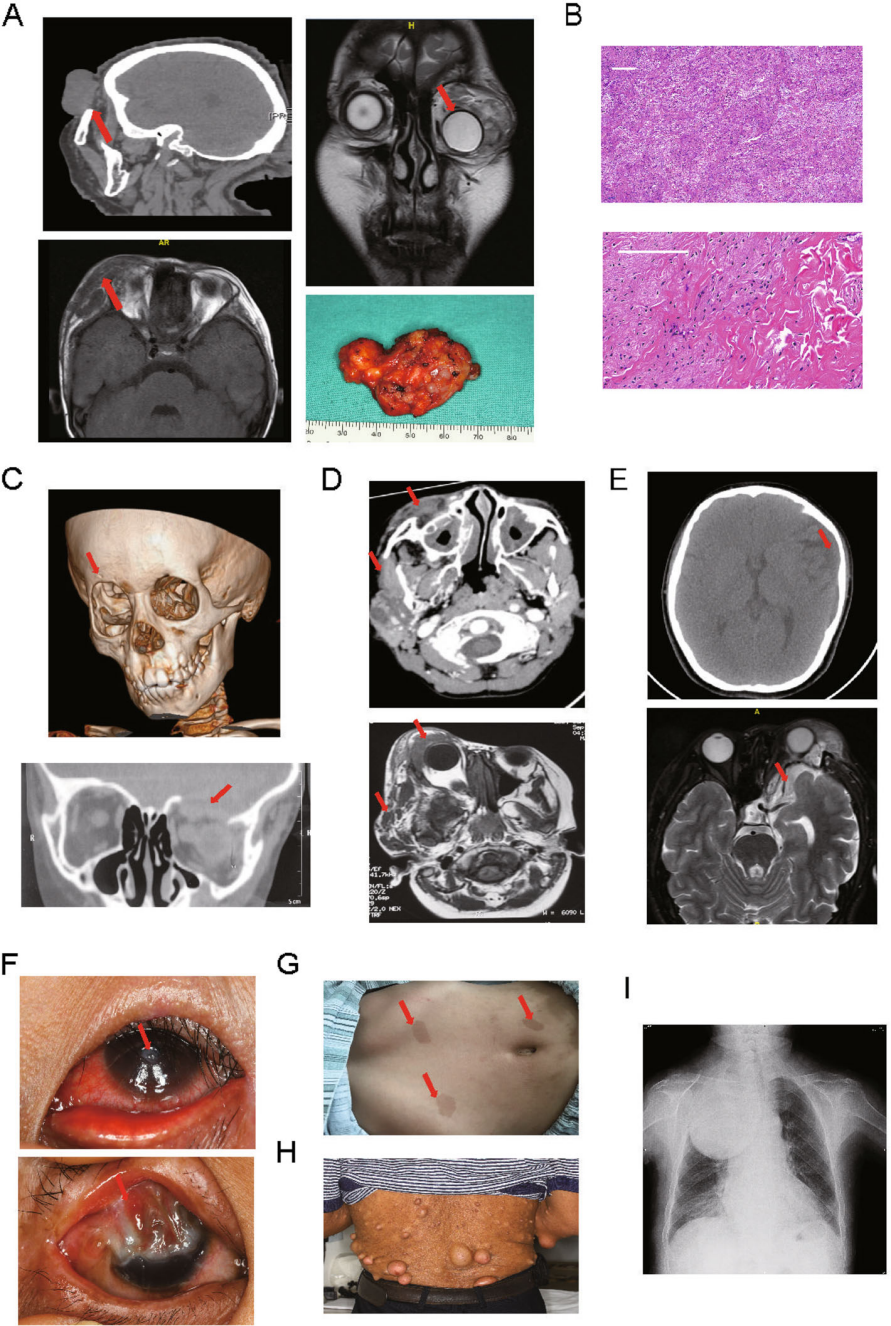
Clinical manifestations of orbital/periorbital plexiform neurofibromatosis (OPPN) patients. a OPPN located in the upper eyelid. Left: Computed tomography (CT) of infiltrative OPPN. Right upper: Magnetic resonance imaging (MRI) of infiltrative OPPN. The arrow indicates the orbital infiltration of OPPN and displacement of the orbital contents. Right below: General view of a dissected OPPN. b Pathological feature of OPPN: abundant collagen fibres and nerve sheaths. Scale bar: 40 μm. c CT images of OPPN resulting in orbital bone destruction. The arrow indicates the area of orbital osteoclasia. d CT images of facial infiltration of OPPN. The arrow indicates the infiltrated area of OPPN. e CT images of intracranial infiltration of OPPN. The arrow indicates the intracranial infiltration. f Upper: corneal ulceration. Below: Surgery-induced symblepharon. g Café au lait spots. h Coexisting extraocular OPPN. i X-ray images showing an enormous mass in the right chest of OPPN patients
